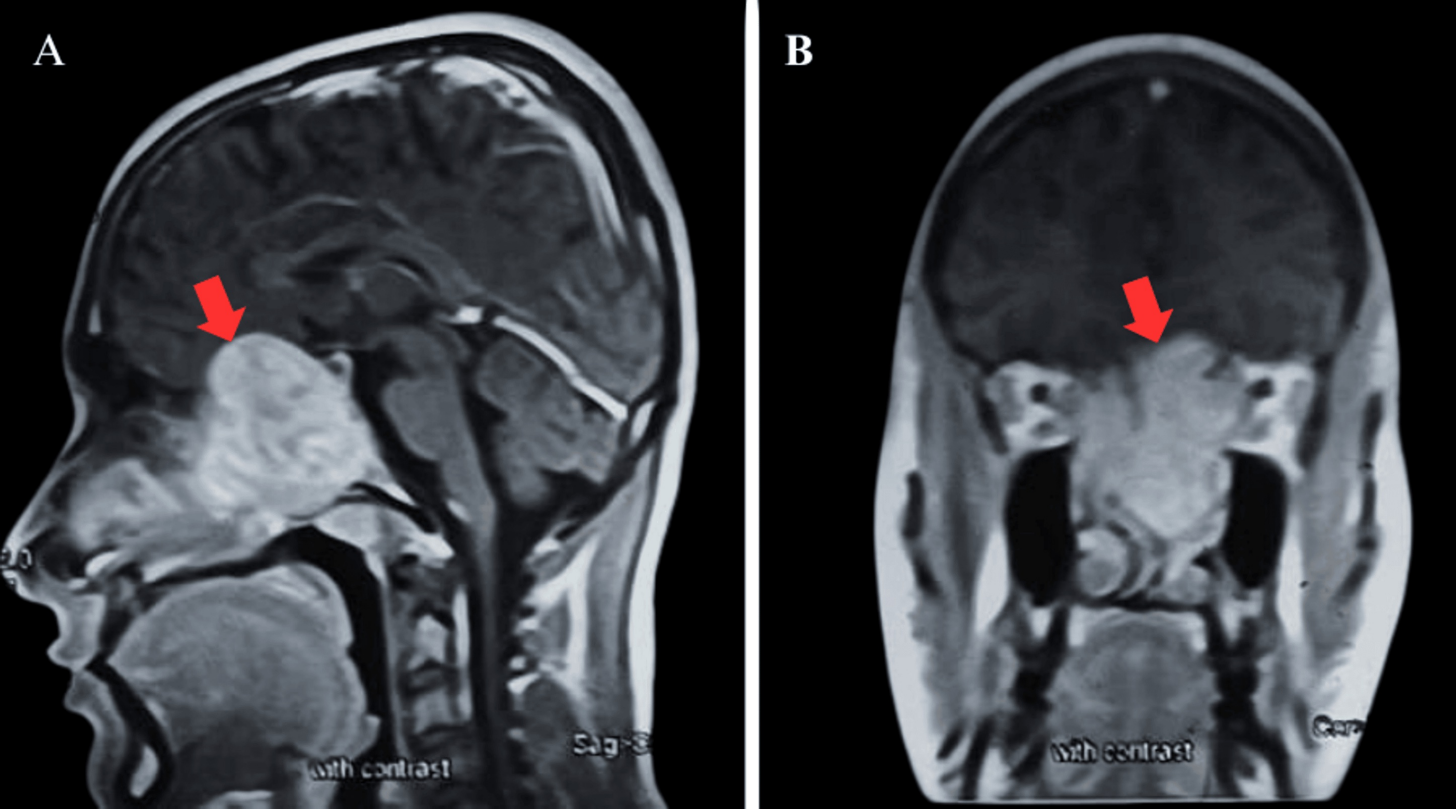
Coronal (A) and sagittal (B) MRI with contrast showing enhancing lesion (red arrows) compressing bilateral optic nerves and reaching orbital apices.
